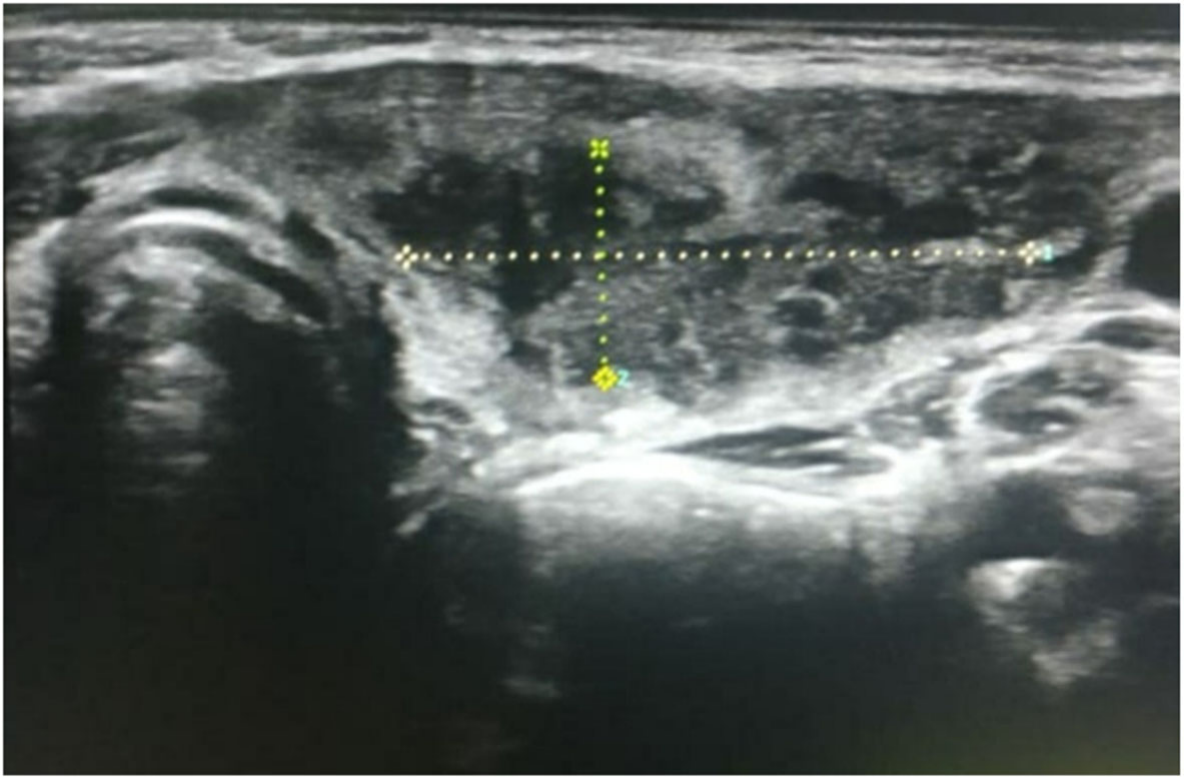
Ultrasonography of neck suggestive of intercommunicating pockets of collections with internal echoes in left thyroid lobe (demarcated dotted area)
